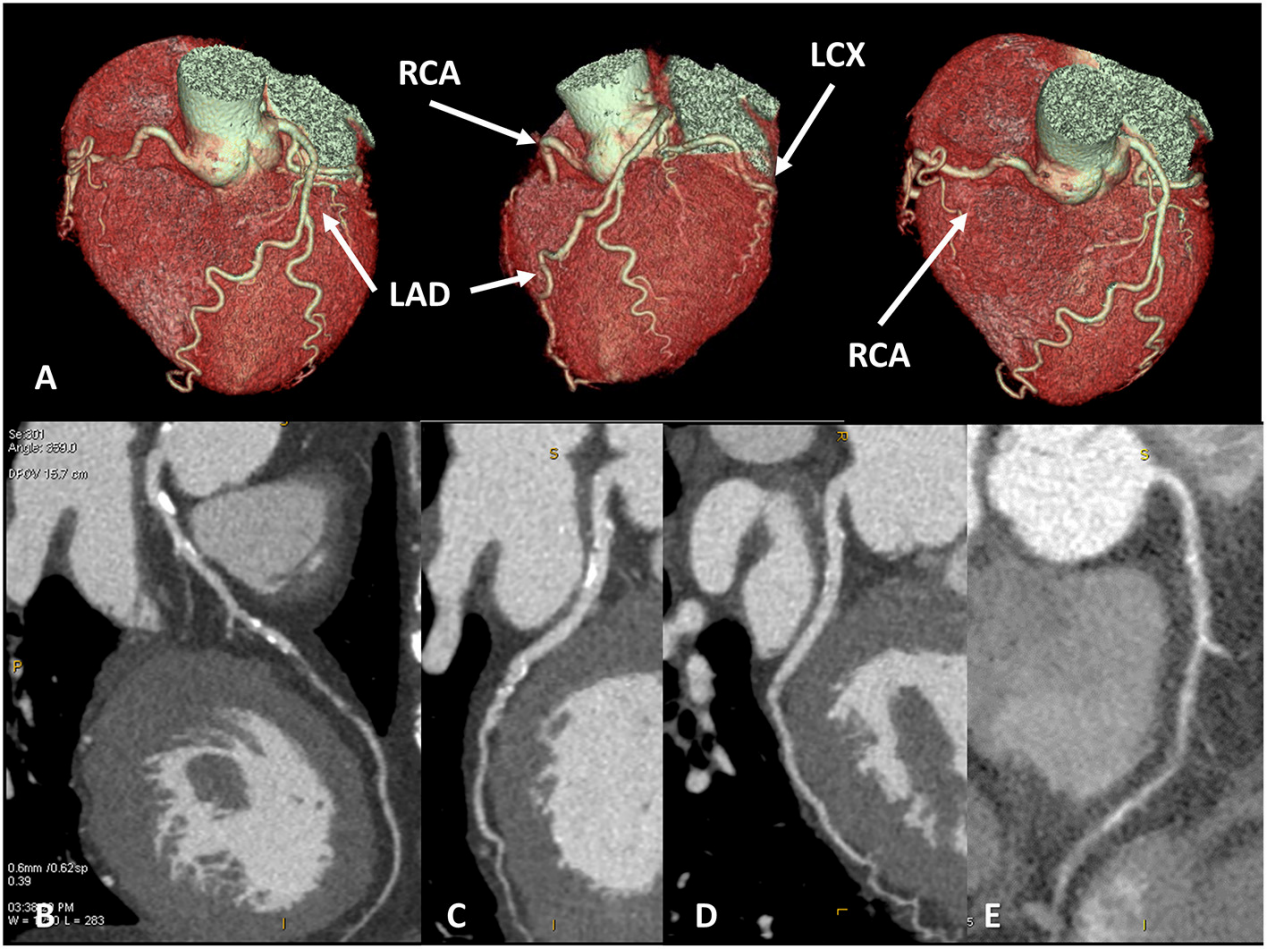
Coronary artery anatomy evaluation. A case example of 65 years old female patients with newly diagnosed severe reduction of left ventricular ejection fraction (<30%), symptomatic for dyspnea. Cardiac CT was performed with optimal image quality (A). A severe multivessel coronary artery disease was found with a subocclusive lesion on mid-left anterior descendent artery (B), significative lesion on left-circumflex artery (C), no significative lesion on the marginal branch and subocclusive disease on right coronary artery (D,E).
